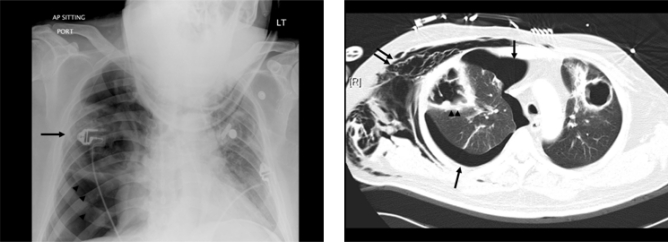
Chest X-ray (A) and contrast-enhanced computed tomography (CT) axial image of the thorax (B) showing right-sided pneumothorax with bilateral thick-walled cavities. The subcutaneous emphysema seen on CT is due to the presence of a right-sided chest drain (not shown).
